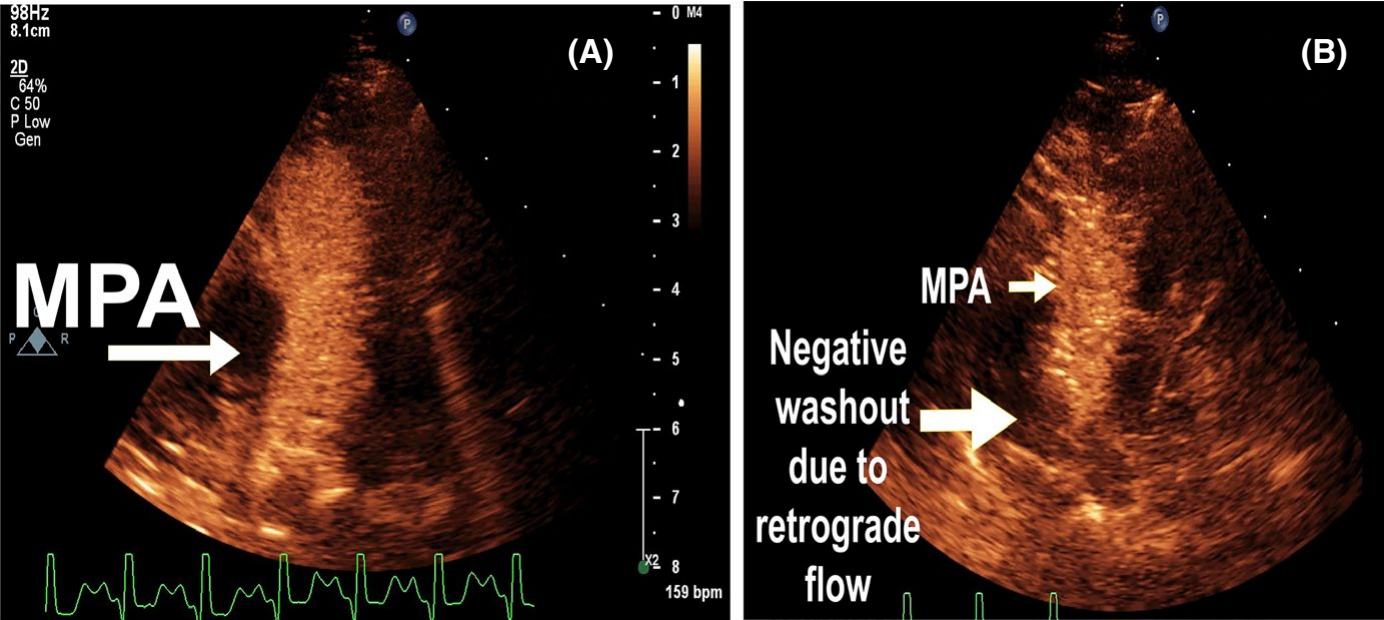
Pulmonary contrast echocardiography. A, Indicates main pulmonary artery filled with microbubbles in the parasternal short‐axis view (color map is turned on for better visualization). B, Shows the negative washout at the location of origin of the anomalous left coronary artery. MPA = main pulmonary artery
